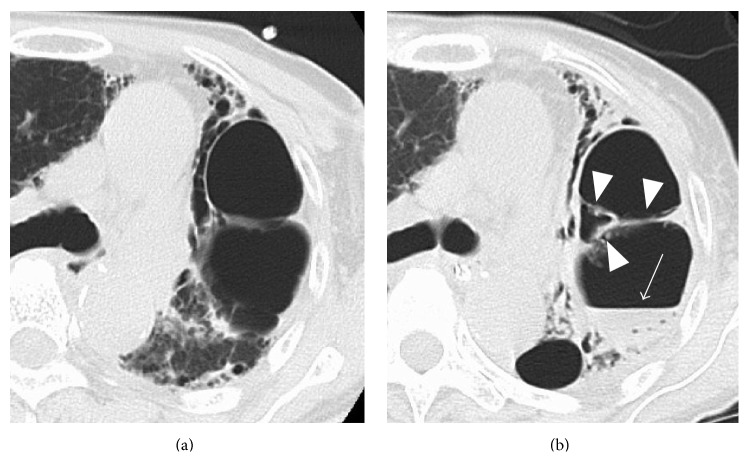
A 70-year-old woman (case 1). (a) Chest CT performed immediately after her arrival at our hospital (60 min after the estimated CAE onset). There is a giant bulla (8 cm in diameter) with septa in the left upper lobe. (b) Chest CT scan obtained 10 days after her admission shows collapse of the bulla with fluid collection (arrow) and thickening of the septa (arrow heads).
